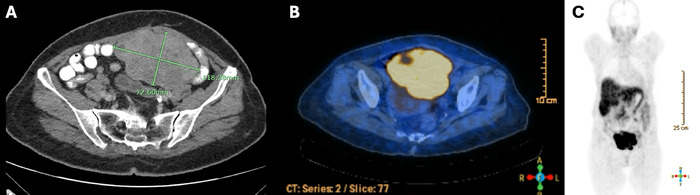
(A) Computed tomography of the abdomen shows a large, lobulated, heterogeneously enhancing mass in the left adnexa measuring 7.3 × 11.8 × 9.0 cm. (B, C) Gallium-68 DOTATATE positron emission tomography/computed tomography demonstrates intense radiotracer uptake (maximum standardized uptake value 48.9) within the adnexal lesion.
